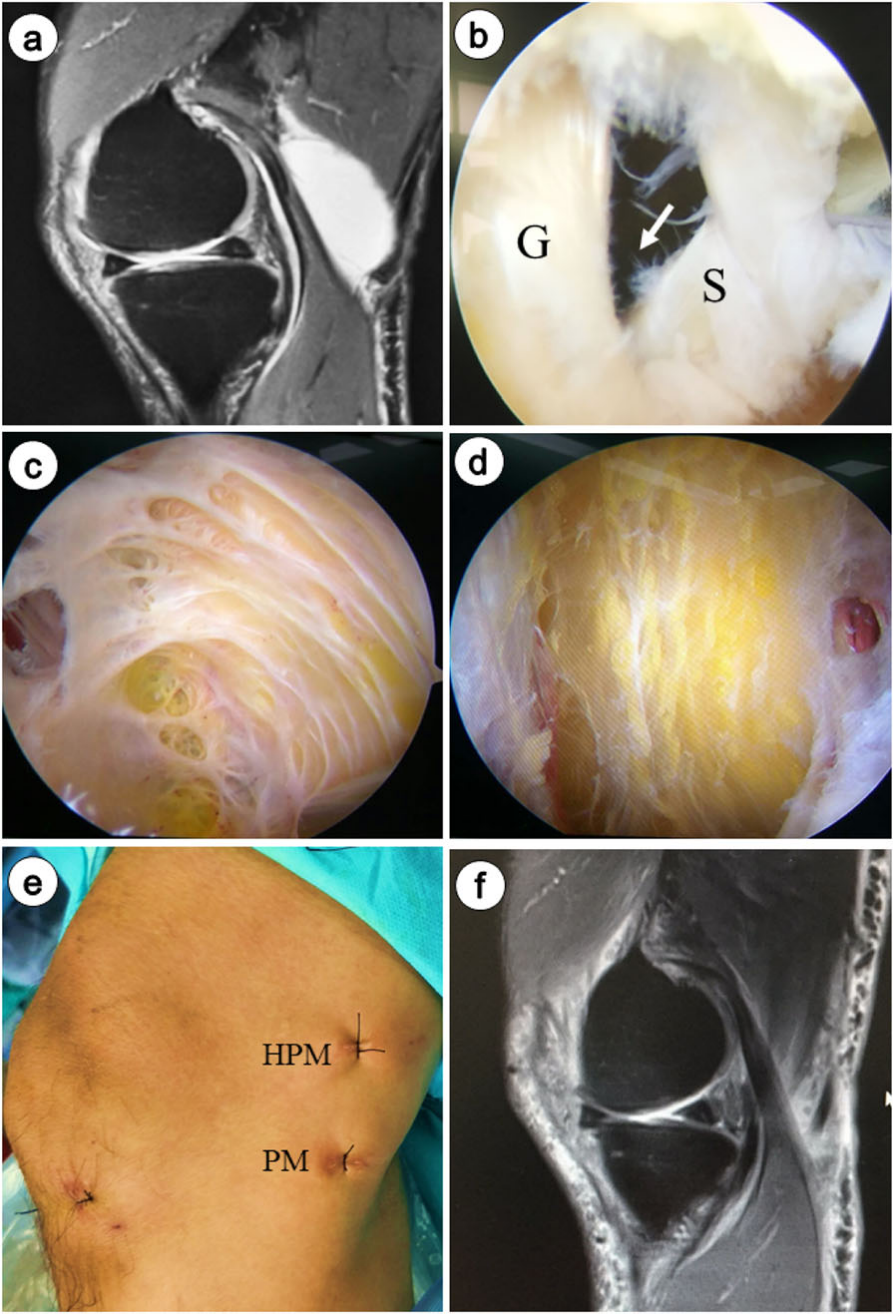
a Pre-operative MR images demonstrate a popliteal cyst on the right knee, b Arthroscopic view of the opening (white arrow) between the popliteal cyst and joint space, after we resected the capsular fold with a shaver and exposed the medial head of gastrocnemius (G) and the semimembranosus tendon (S), c Arthroscopic view of the inner cystic wall of the popliteal cyst via posteromedial portal, d Arthroscopic view after the cyst wall excision was completed, e Postoperative photograph showing external landmarks of posteromedial (PM) portal and high posteromedial (HPM) portal, f Last follow-up (24 months after surgery) MR images demonstrate no recurrence of the popliteal cyst on the right knee
